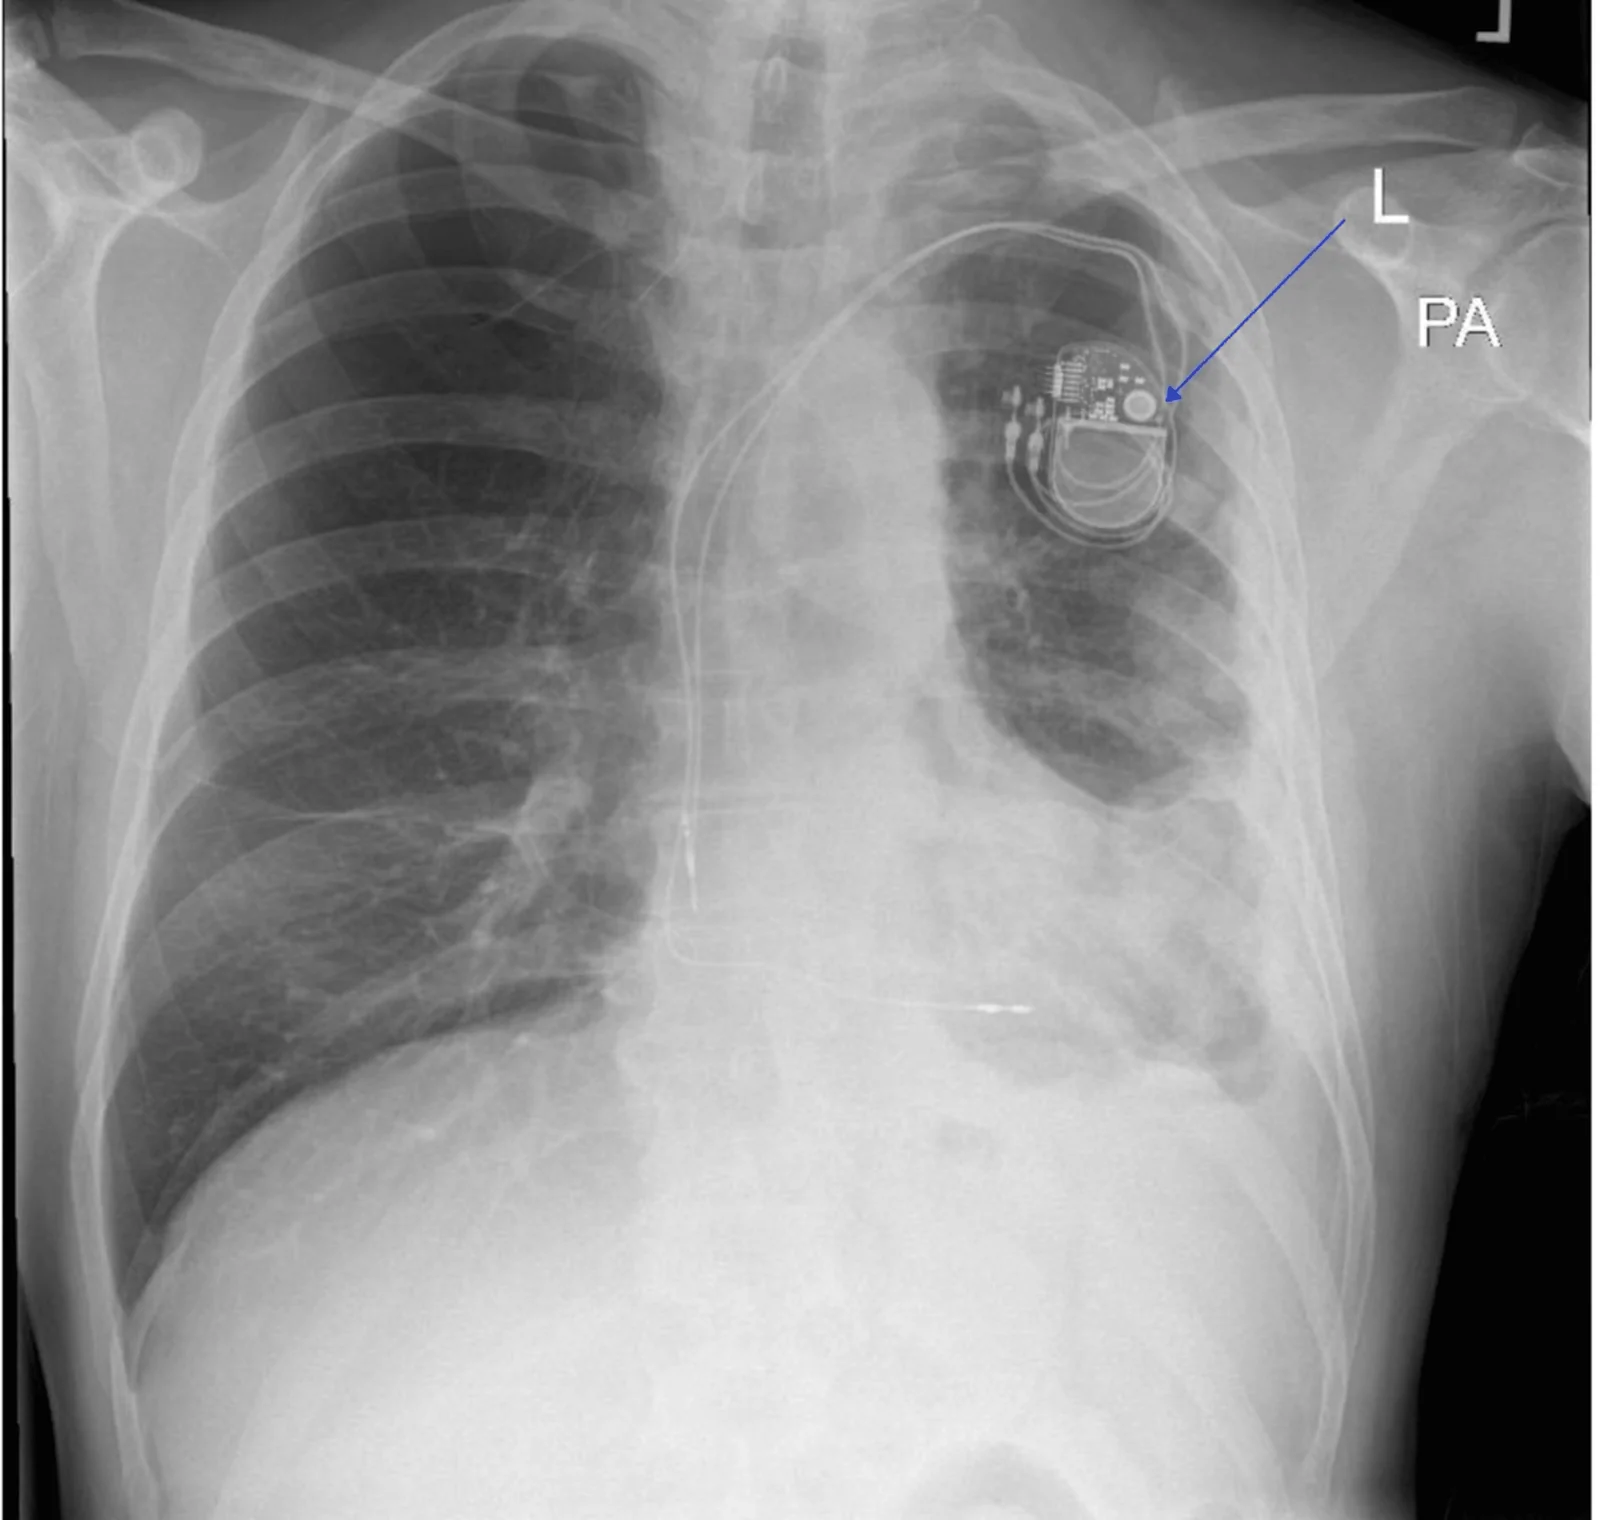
Chest X-ray showing a well-positioned dual chamber pacemaker in the left pectoral region (blue arrow)
